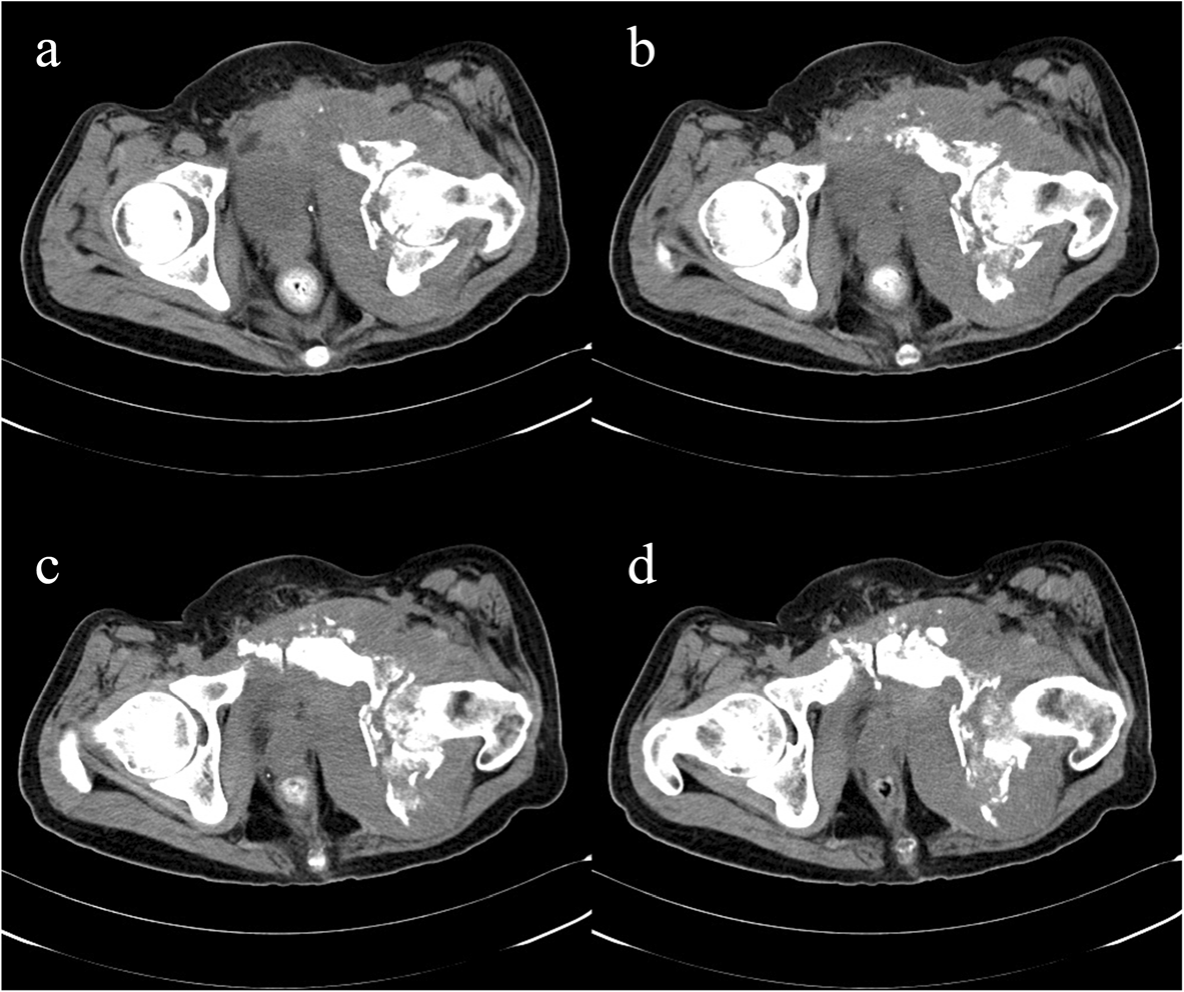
CT indicates that the sigmoid colon carcinoma metastasizes to the bone and soft tissues. a. The surrounding soft tissues of the left hip joint swell significantly and the spatium intermusculare disappears. b. The surrounding soft tissues of the left hip joint swell significantly and the spatium intermusculare disappears. c. Left acetabulum and pubic symphysis appear multiple bone destructions. d. Left acetabulum and pubic symphysis appear multiple bone destructions.
